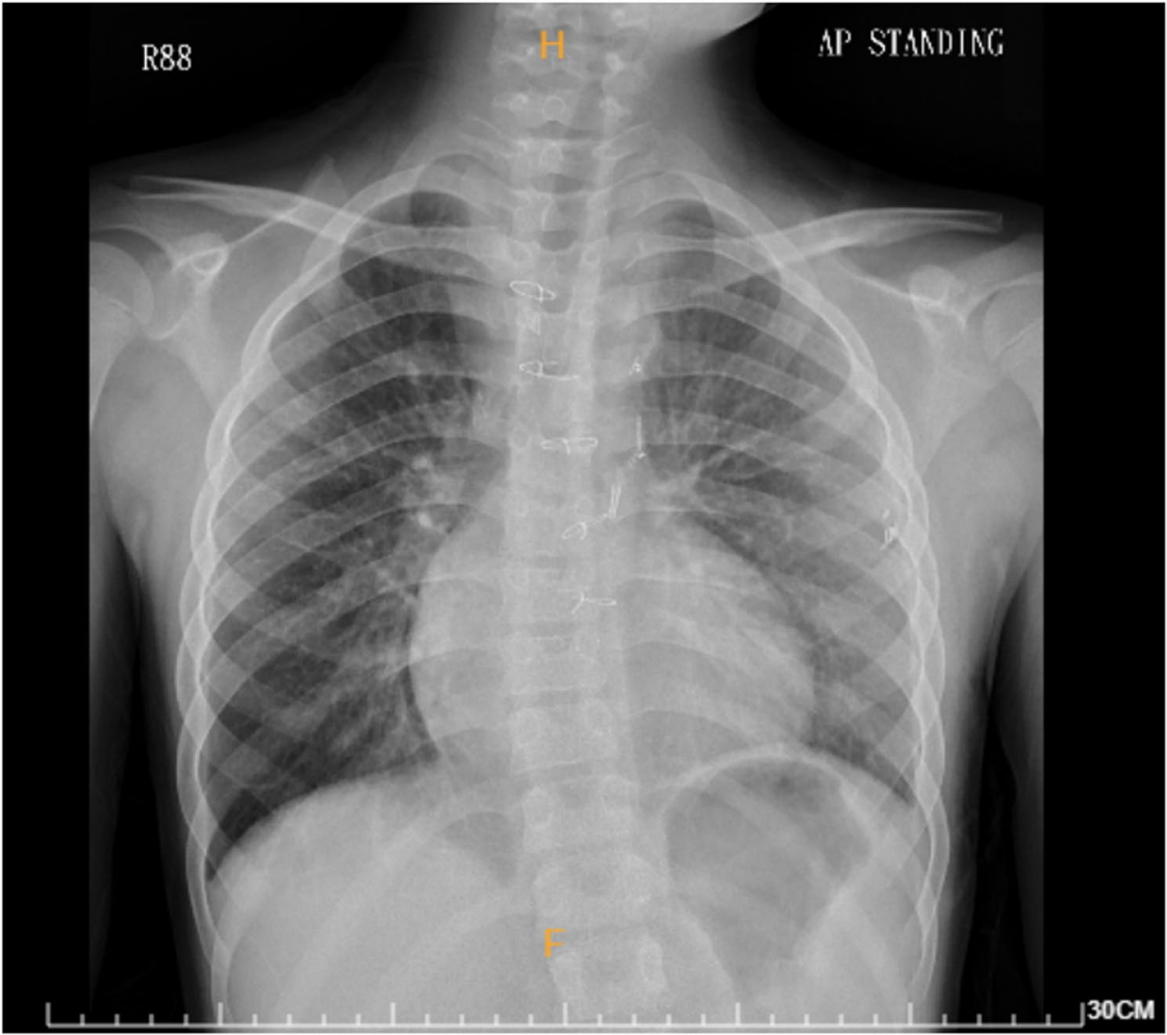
A chest radiograph taken 2 months before the current hospitalization showing enlargement of the cardiac silhouette on the right side
